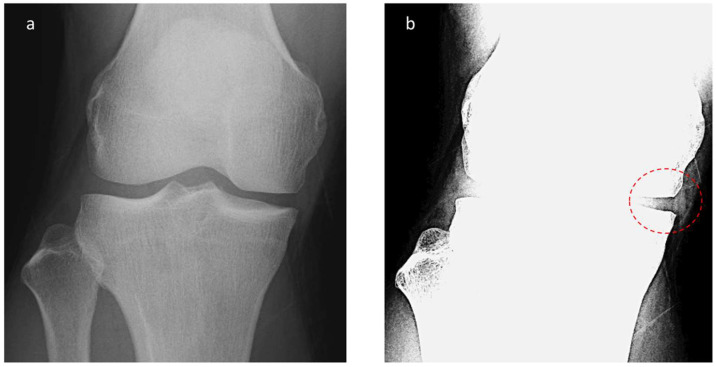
Radiographic appearance of meniscal extrusion. (a) Anteroposterior (AP) view knee radiograph with standard window settings. (b) AP knee radiograph with narrow window settings demonstrates visualized meniscal tissue.
